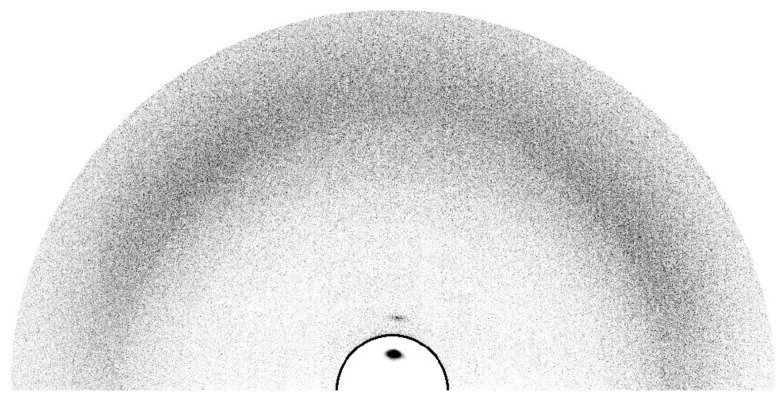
Grazing incidence X-ray pattern at 100 °C on the top of a 3_12_ droplet, slowly cooled down from isotropic phase; for image contrast compatibility with the surrounding zone, intensities inside the zone, delimited by the black line and containing the first order reflection of the lamellar stacking, are divided by one hundred.
